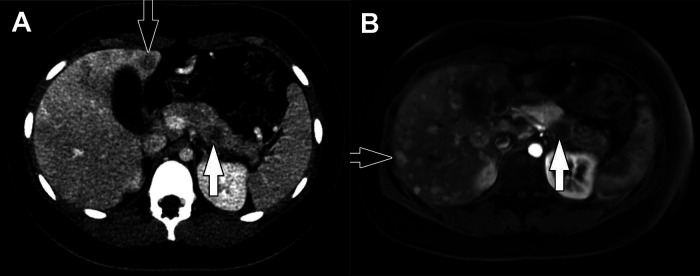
Insulinoma. (A) Axial CT of the abdomen obtained in the portal venous phase following intravenous contrast material administration shows a hypoenhancing mass in the body of the pancreas (white arrow) with multiple hypoenhancing liver metastases (one indicated by the black arrow). Characteristically, neuroendocrine tumors hyperenhance in the arterial phase following contrast administration but hypoenhancing lesions do occur. (B) Axial T1-weighted fat-saturated post-contrast MRI obtained in the arterial phase shows the pancreatic tumor (white arrow) to be hypoenhancing while the multiple liver metastases (one indicated by the black arrow) are hyperenhacing. The tail of the pancreas upstream of the tumor is hypoenhancing due to obstructive pancreatitis related to the tumor.
